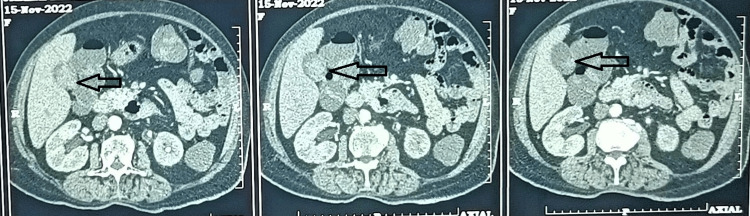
Contrast-enhanced computed tomography (CECT) (AP) showing polypoidal lesion (arrows)
